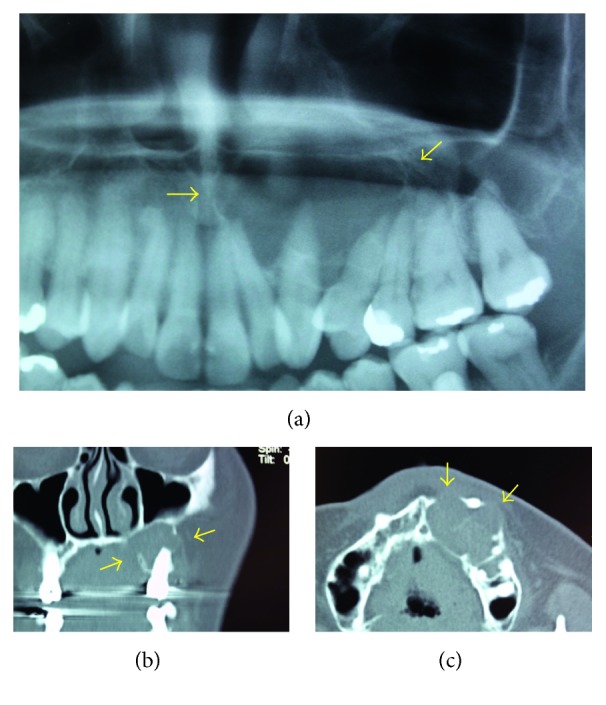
Radiologic and computed tomography (CT) findings. (a) Panoramic radiograph showing a well-delineated radiolucent lesion (yellow arrows). (b, c) CT showing expansion and cortical bone osteolysis (yellow arrows).
